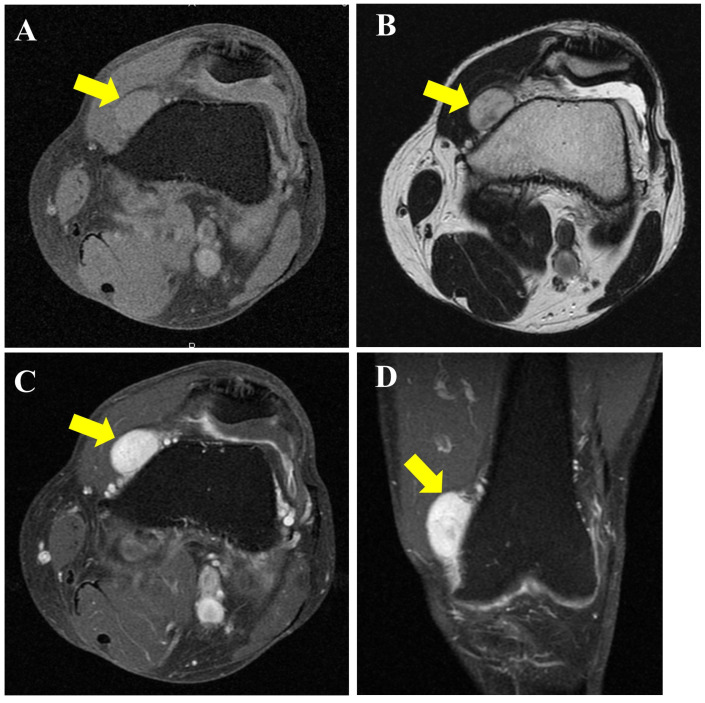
Magnetic resonance imaging (MRI) findings demonstrated an intra-articular lesion (yellow arrows). The tumor showed isointensity relative to muscle on T1-weighted fat-suppressed images (A), heterogeneous high signal intensity on T2-weighted images (B), and marked enhancement on contrast-enhanced T1-weighted images (C,D). Based on these MRI findings, the differential diagnosis included vascular and perivascular tumors (such as angioleiomyoma and myopericytoma), schwannoma, vascular malformation, and synovial sarcoma. To obtain a definitive diagnosis, an incisional biopsy was performed, which suggested a pericytic tumor, such as myopericytoma. The lesion was excised at the patient’s request to alleviate pain.
